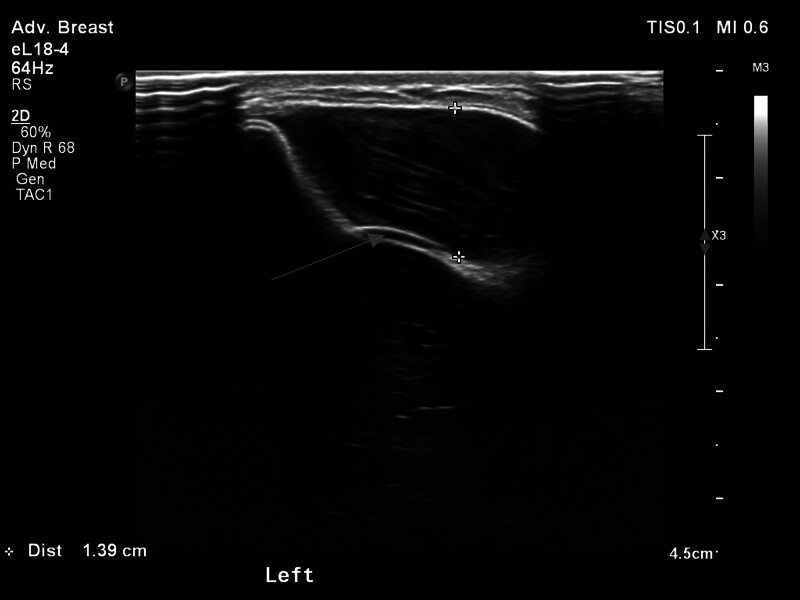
Breast ultrasound revealed capsule morphology with a surrounding hypoechoic fluid collection, accompanied by suspicious high echogenic linear signal beneath the capsule (indicated by the red arrow).
